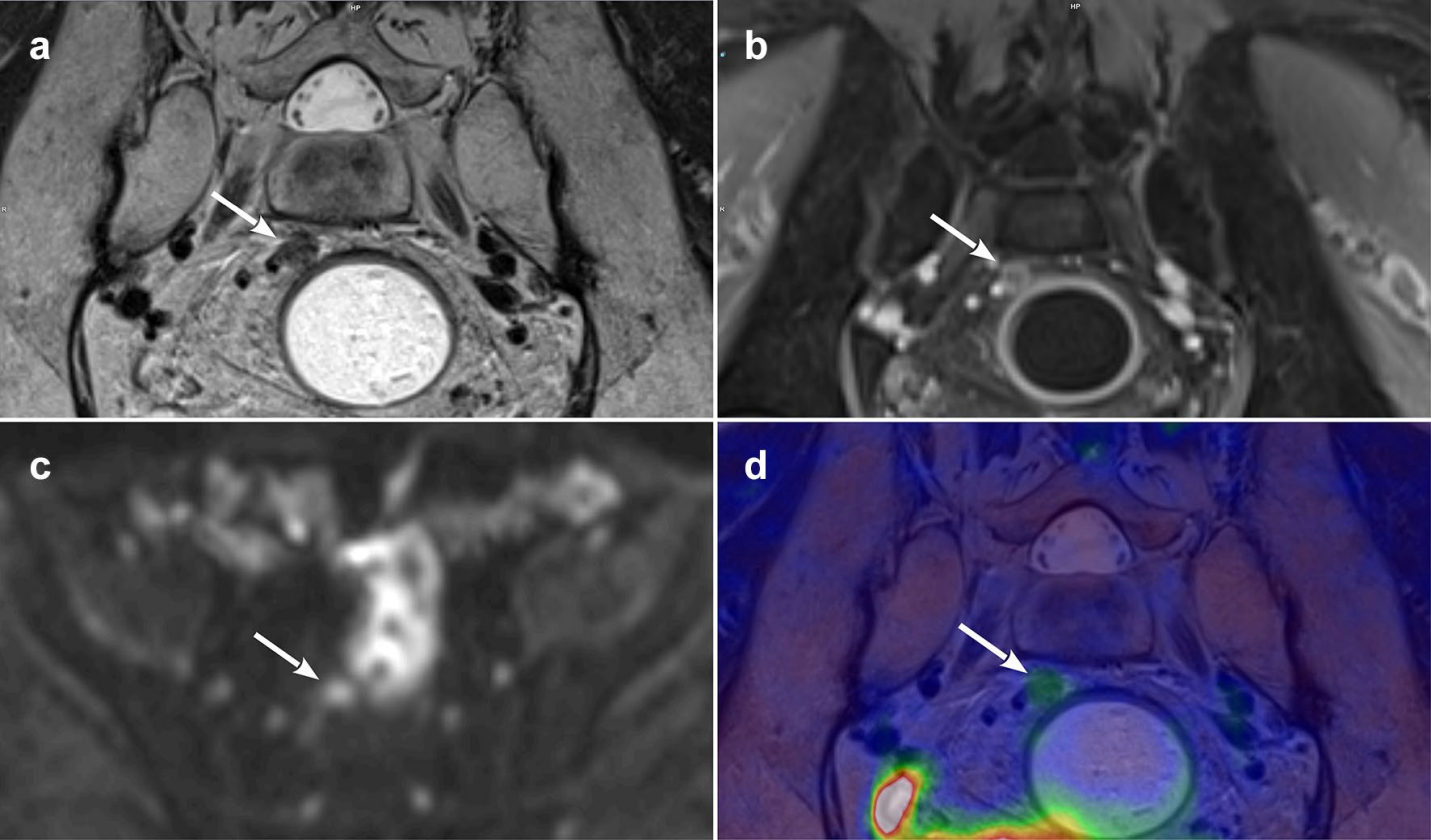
PET/MR images of a malignant mesorectal lymph node after pCRT. a
